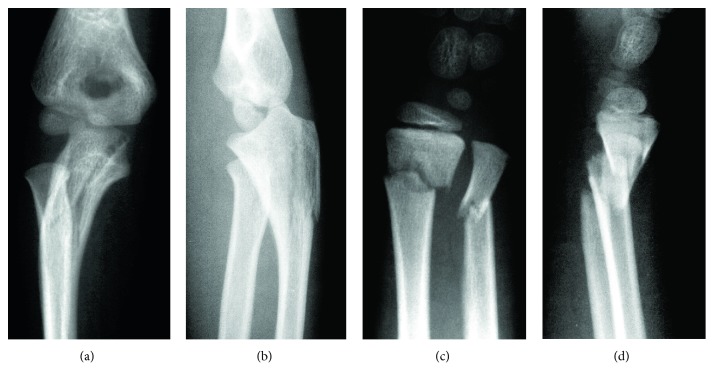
X-rays images after manual reduction. (a) AP view of the elbow joint showing subluxation of the radial head. (b) Lateral view of the elbow joint. (c) Front view of the wrist joint showing dislocation of the distal radius. (d) Lateral view of the wrist joint showing dislocation of the distal radius.
